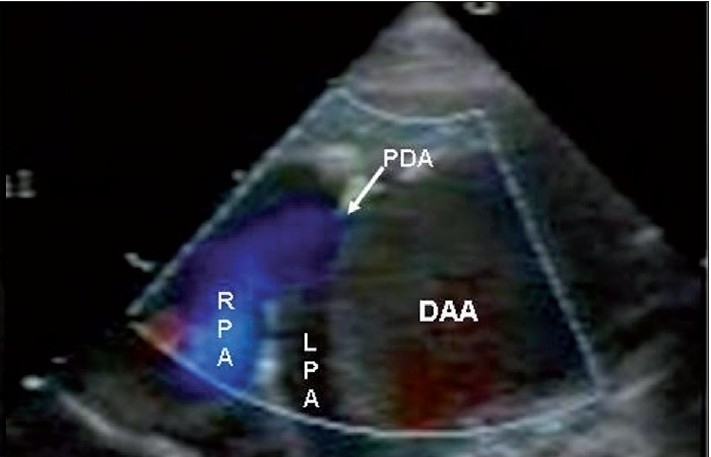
High parasternal short axis view with color flow mapping showing tiny ductal flow (left to right) with normal flow in the right pulmonary artery DAA-ductus arteriosus aneurysm, RPA-right pulmonary artery, LPA-left pulmonary artery, DAo-descening aorta. Ao arch-aortic arch, PDA-patent ductus arteriosus
